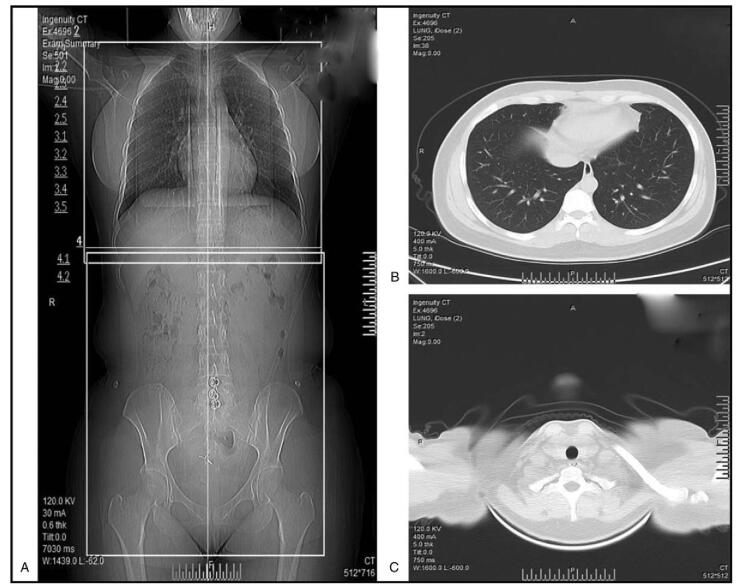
Computed tomography (CT) imaging of patients diagnosed with PMYST before surgery and postoperative chemotherapy (Case 5). (A) Computed tomography scan on admission. (B) Computed tomography scan of the thorax. (C) Computed tomography scan of the pelvic cavity.
